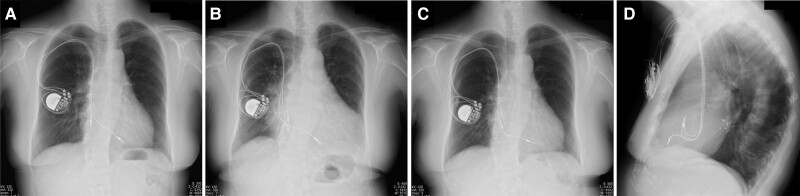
Chest X-ray images after pacemaker implantation (*A*), when heart failure developed 6 years later due to pacing-induced cardiomyopathy (*B*), and 6 months after cardiac resynchronization therapy pacemaker upgrade in frontal view (*C*) and lateral view (*D*). CS, coronary sinus; CRT-P, cardiac resynchronization therapy pacemaker; PLSVC, persistent left superior vena cava.
